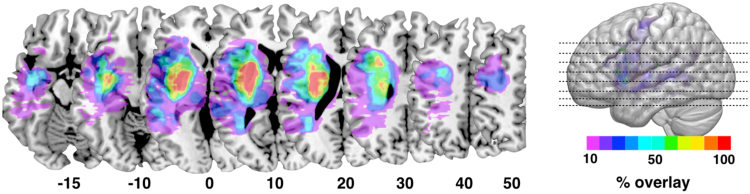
Percentage lesion overlay maps based on lesion masks delineated on T1-weighted images.
